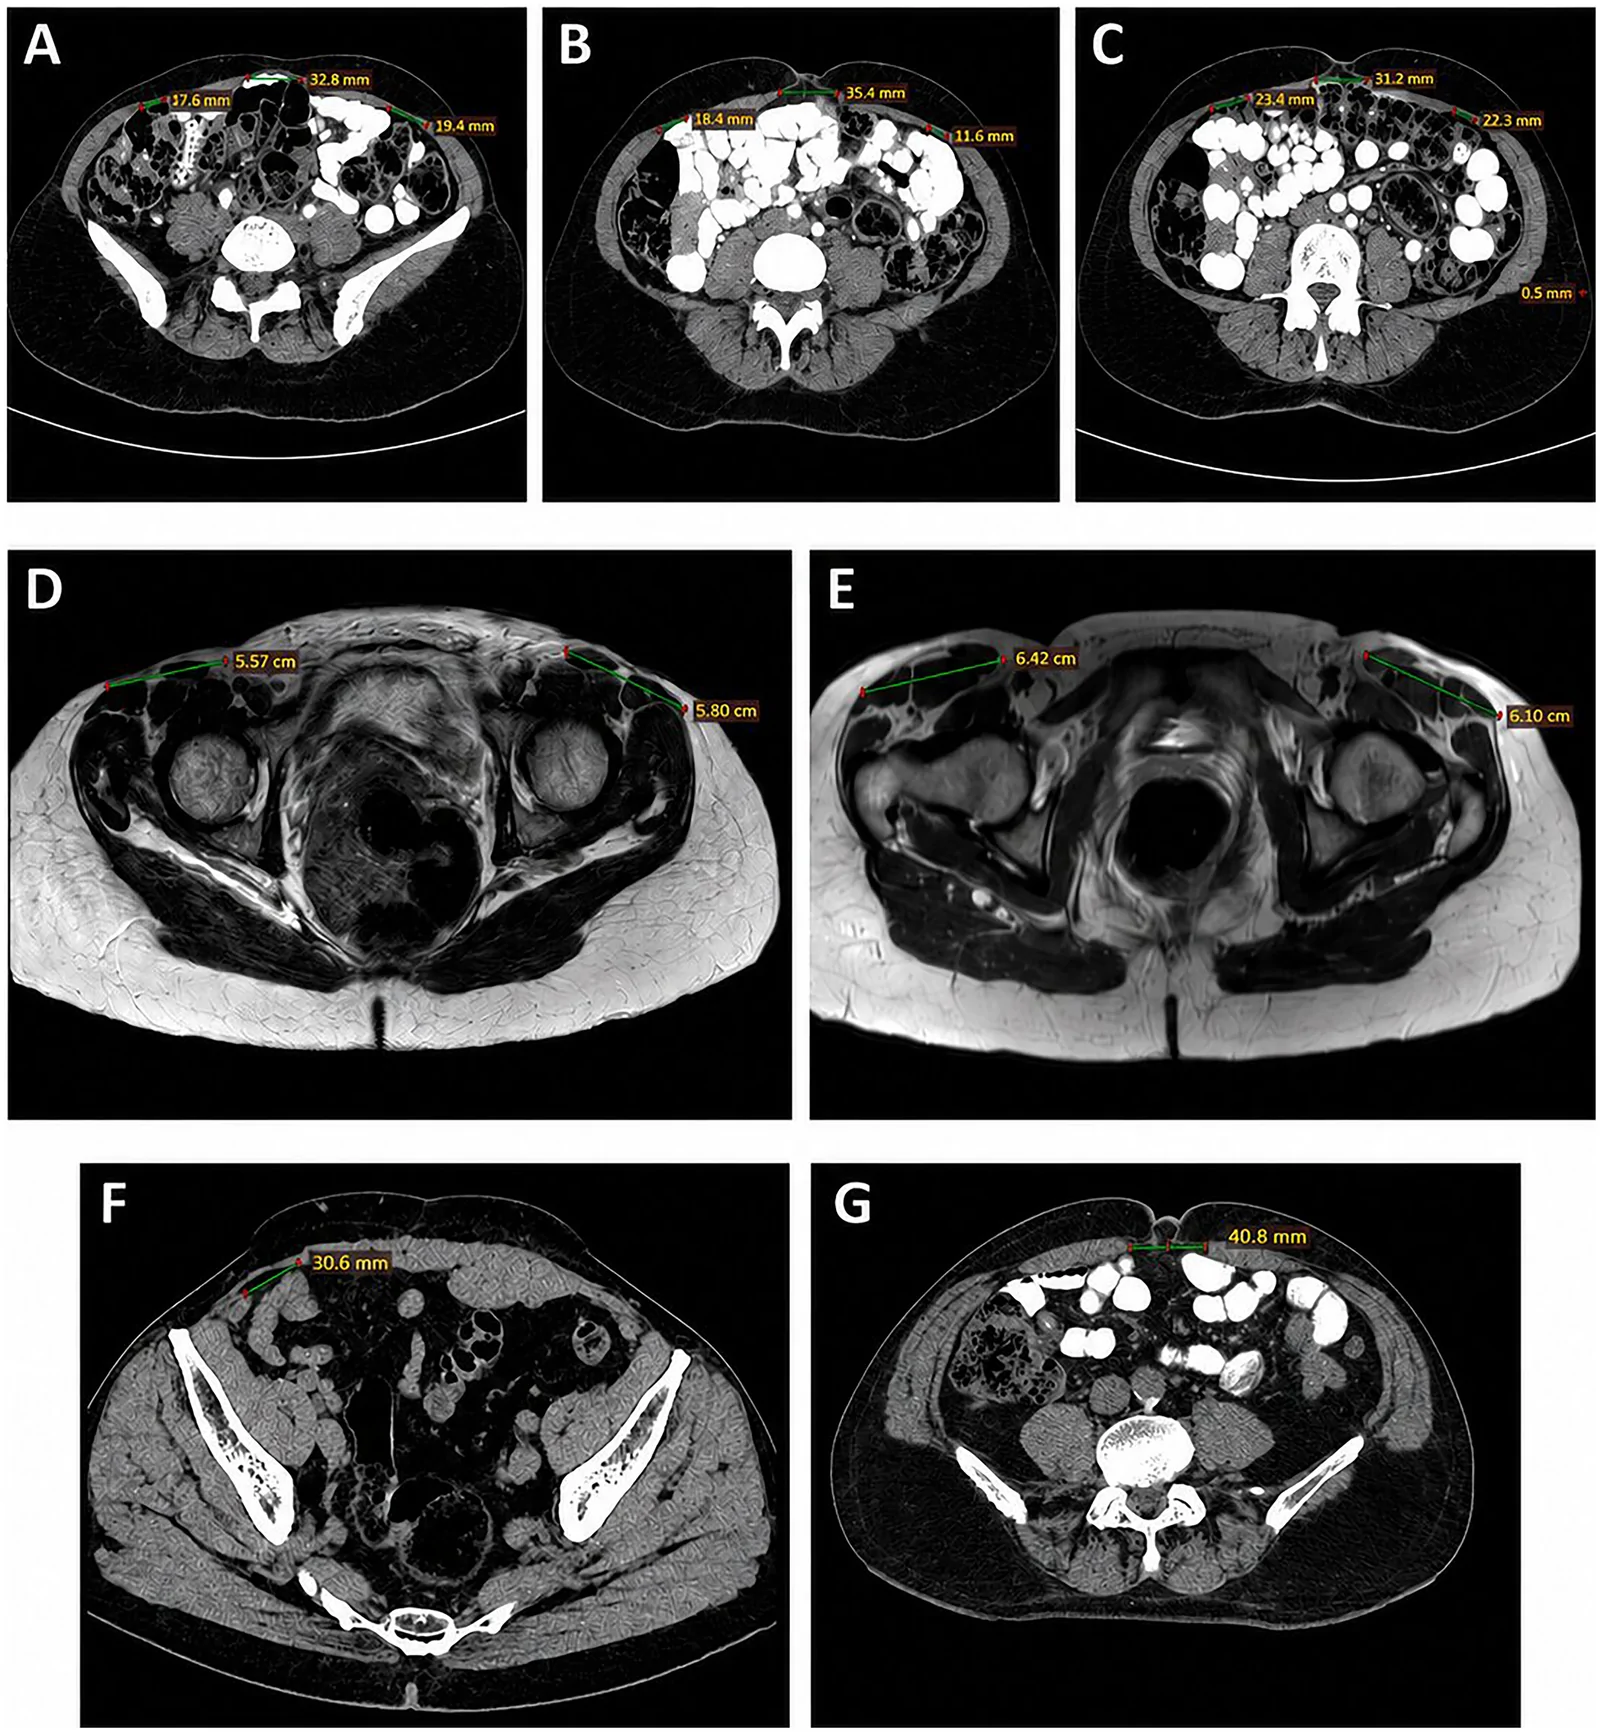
Representative pre- and postoperative cross-sectional imaging from two early cases treated with isolated midline RD repair. (A–C) Preoperative axial CT images from Case 1 at three abdominal levels, demonstrating RD with minimal BSLD. (D,E) Postoperative axial MRI images from the same patient, demonstrating marked bilateral SLD on follow-up imaging. (F,G) Pre- and postoperative axial CT images from Case 2, respectively, demonstrating an increase in right-sided SLD from 1.3 cm preoperatively to 3.6 cm postoperatively following RD repair, without a comparable increase on the left. The overlaid calipers indicate the measured RD and SLD widths at the respective axial levels.
